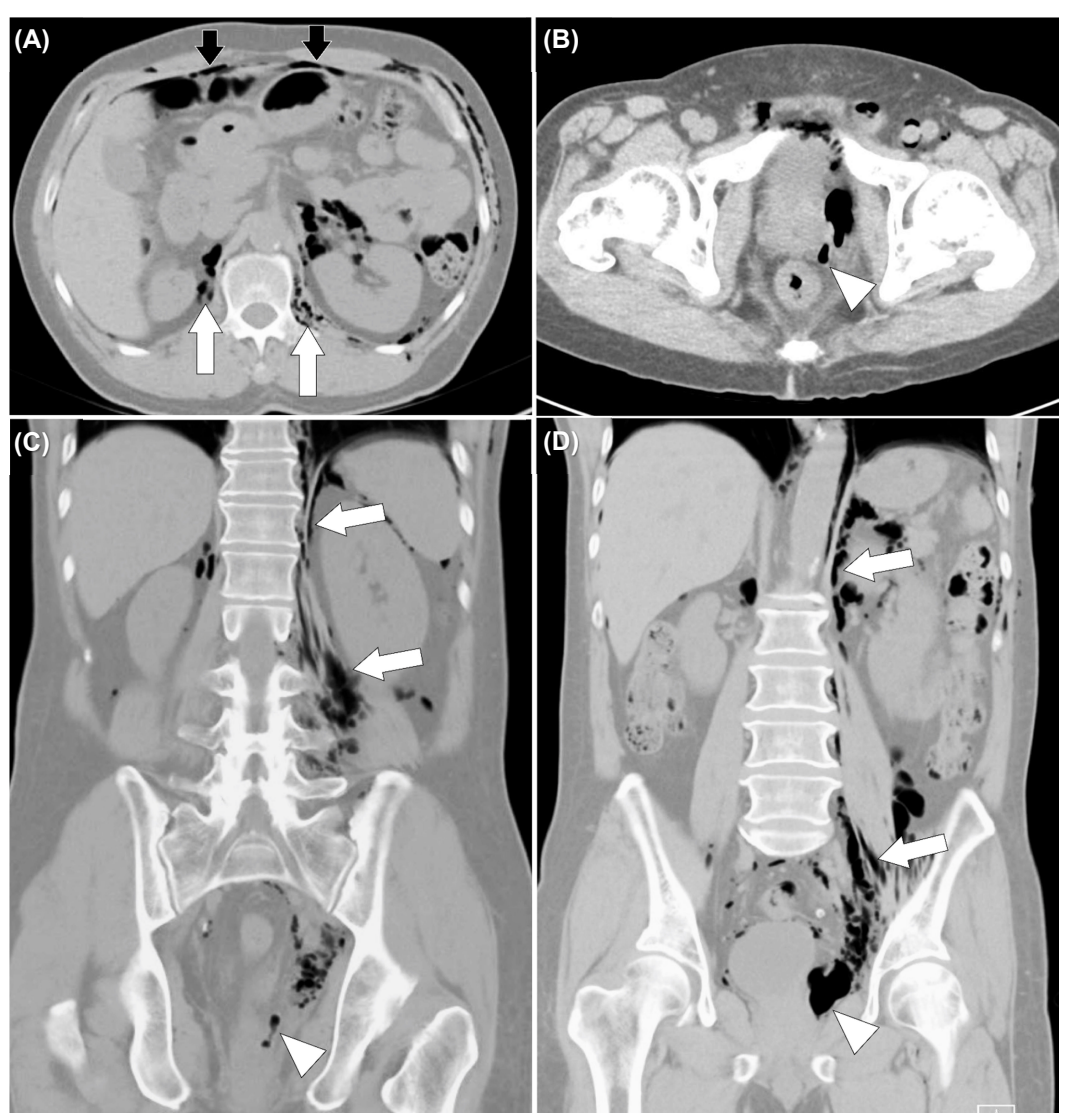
Non-enhanced computed tomography of the abdomen showed air tract extended from left peri-rectal area (white arrowheads) (B–D) to retroperitoneum (white arrows) (A,C,D), and peritoneum (black arrows) (A).
